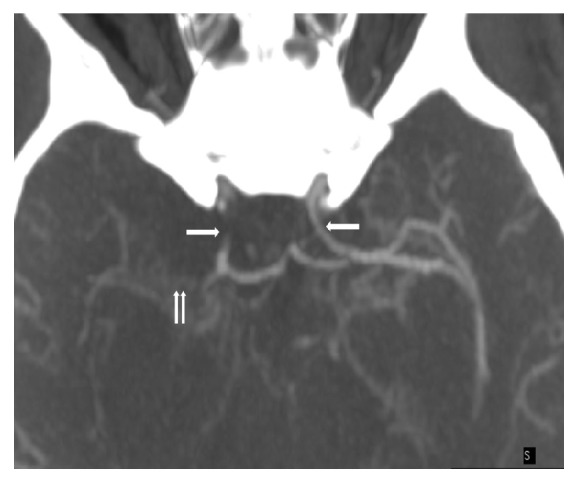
Maximum intensity projection (MIP) axial image of the CT angiogram demonstrates severe stenosis of the internal carotid arteries (single arrows) and occlusion of the Rt, MCA (double arrows).
